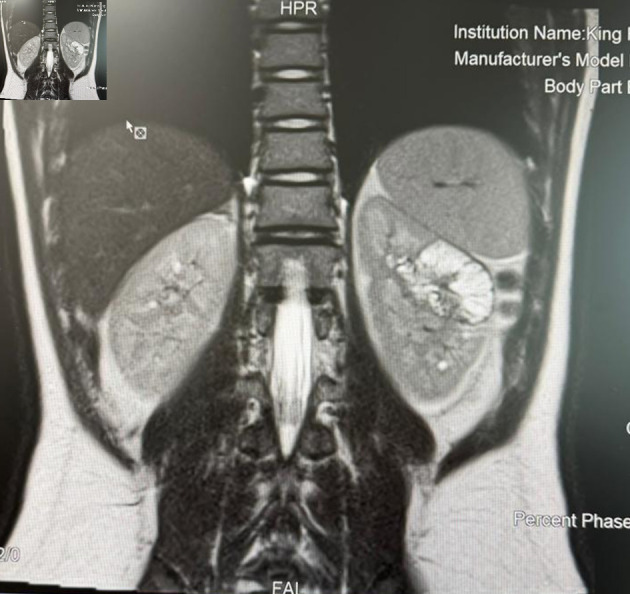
MRI showing a left kidney mass (arrow). MRI: magnetic resonance imaging.
